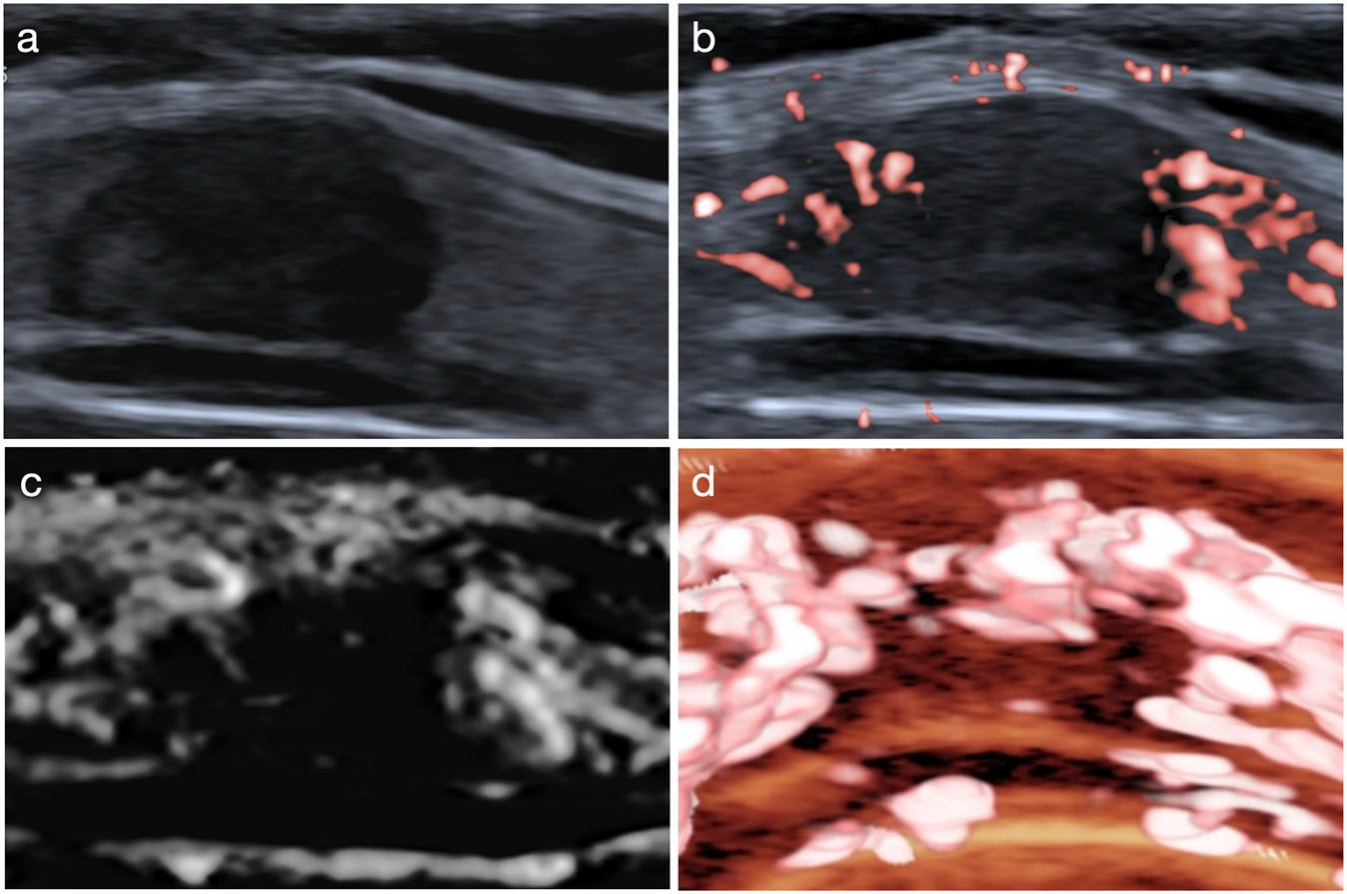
Ultrasound images of a solid hypoechoic nodule of 1.1 cm in the thyroid isthmus of a 41-year-old female patient. After adjustment by the crab claw-like microflow pattern on SMI, one-level upgrade was made for ACR TI-RADS, ATA RSS, KSThR TIRADS, EU-TIRADS and C-TIRADS, and the biopsy recommendations of ACR TI-RADS and EU-TIRADS were added. Postoperative pathology confirmed it was a papillary thyroid carcinoma. a Greyscale ultrasound, b CSMI, c MSMI, d Smart-3D
